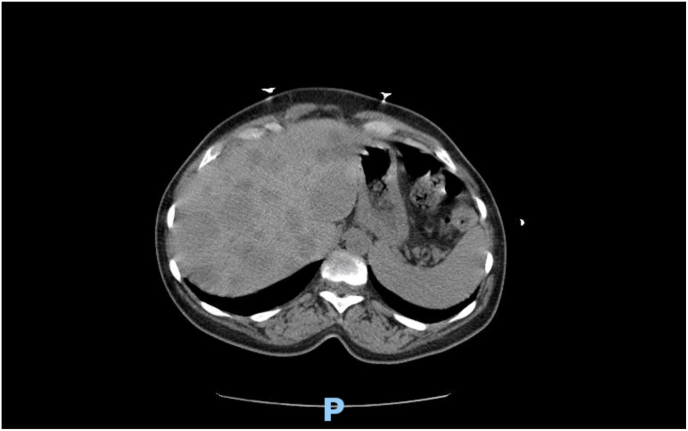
Axial plane of CT scan of abdomen.
